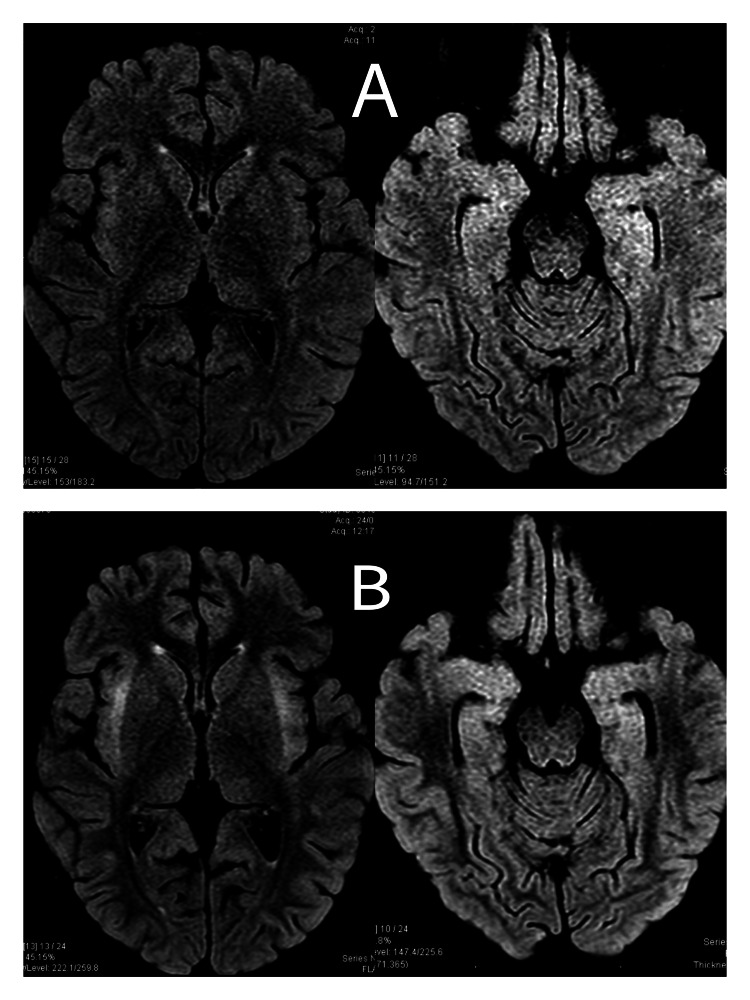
FLAIR MRI sequences prior (A) and after (B) IV steroid treatment. A) MRI prior to the start of IV steroid treatment: Left shows discrete diffuse volume loss is observed. In addition, right shows hyperintensity in both temporal poles and at the right insular and left parietal level (right). B) MRI after completing 10 days of treatment with IV steroids. Left shows insular, external capsule, and claustrum signal change is observed, and the volume loss appears to be increased after the steroid treatment. Right shows evidence of an increased signal in both insulae and medial temporal lobes. In addition, it has no enhancement with gadolinium and does not restrict diffusion sequences. FLAIR: fluid-attenuated inversion recovery
